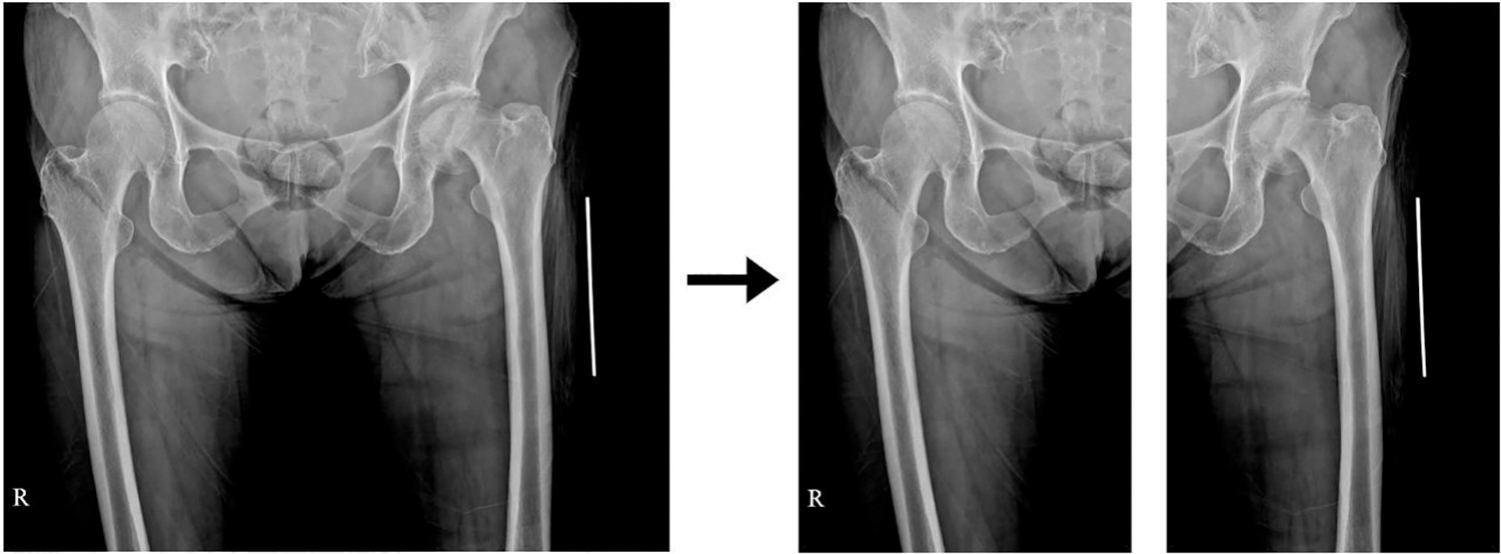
Example of an original hip radiograph presented asymmetrically on the horizontal axis. As the center of the body was not positioned at the exact center of the radiographic image, this caused the image to be skewed to one side when it was cut in half.
